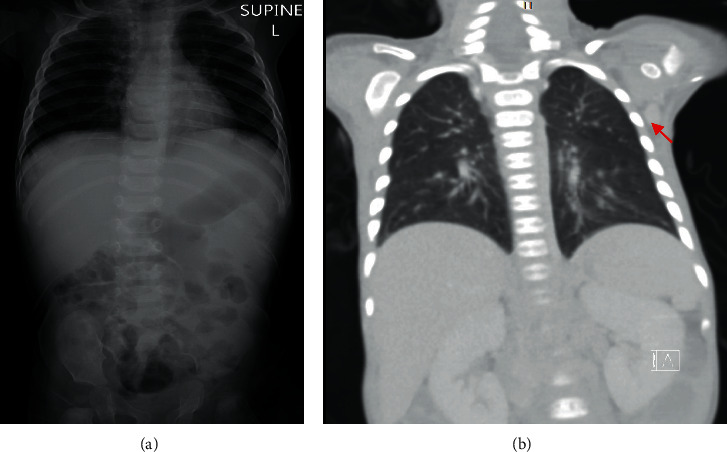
Panel A* (4½ months of age): chest radiograph showing bilateral lung infiltrates and absent thymic shadow. *Panel B* (5 months of age): chest CT scan showing enlarged left axillary lymph node, measuring about 2 cm in diameter (arrowed).
